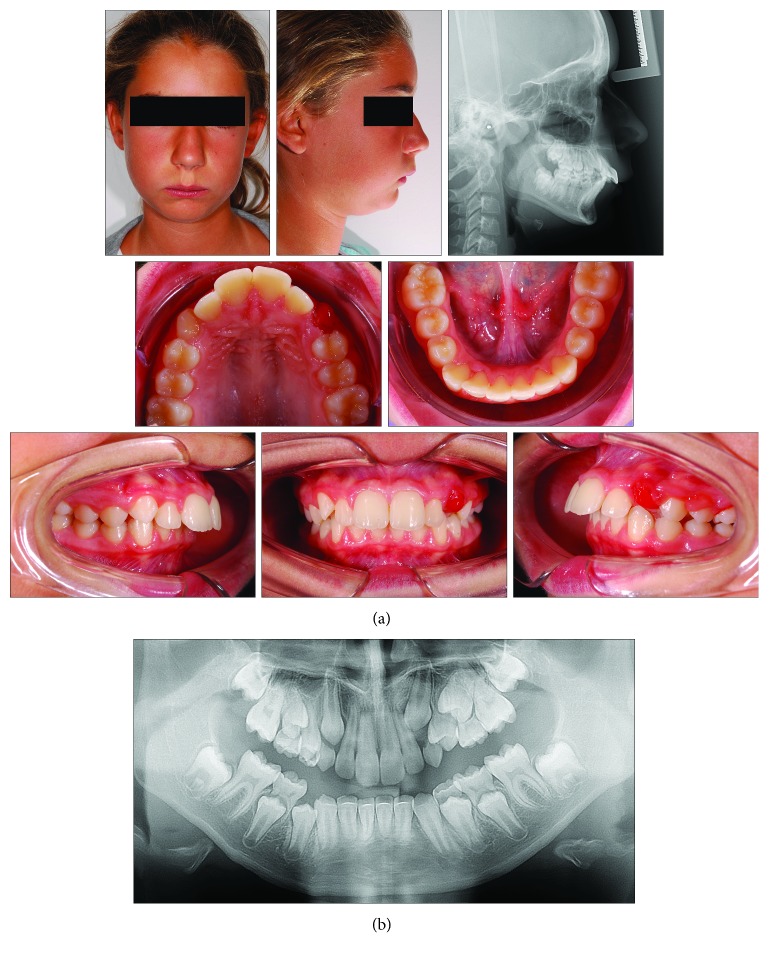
Case 2. (a) 9-year and 8-month-old female patient with skeletal Class II malocclusion, increased overjet, and overbite before treatment. (b) Panoramic radiograph taken at first visit when the patients was 8 years 10 months old.
